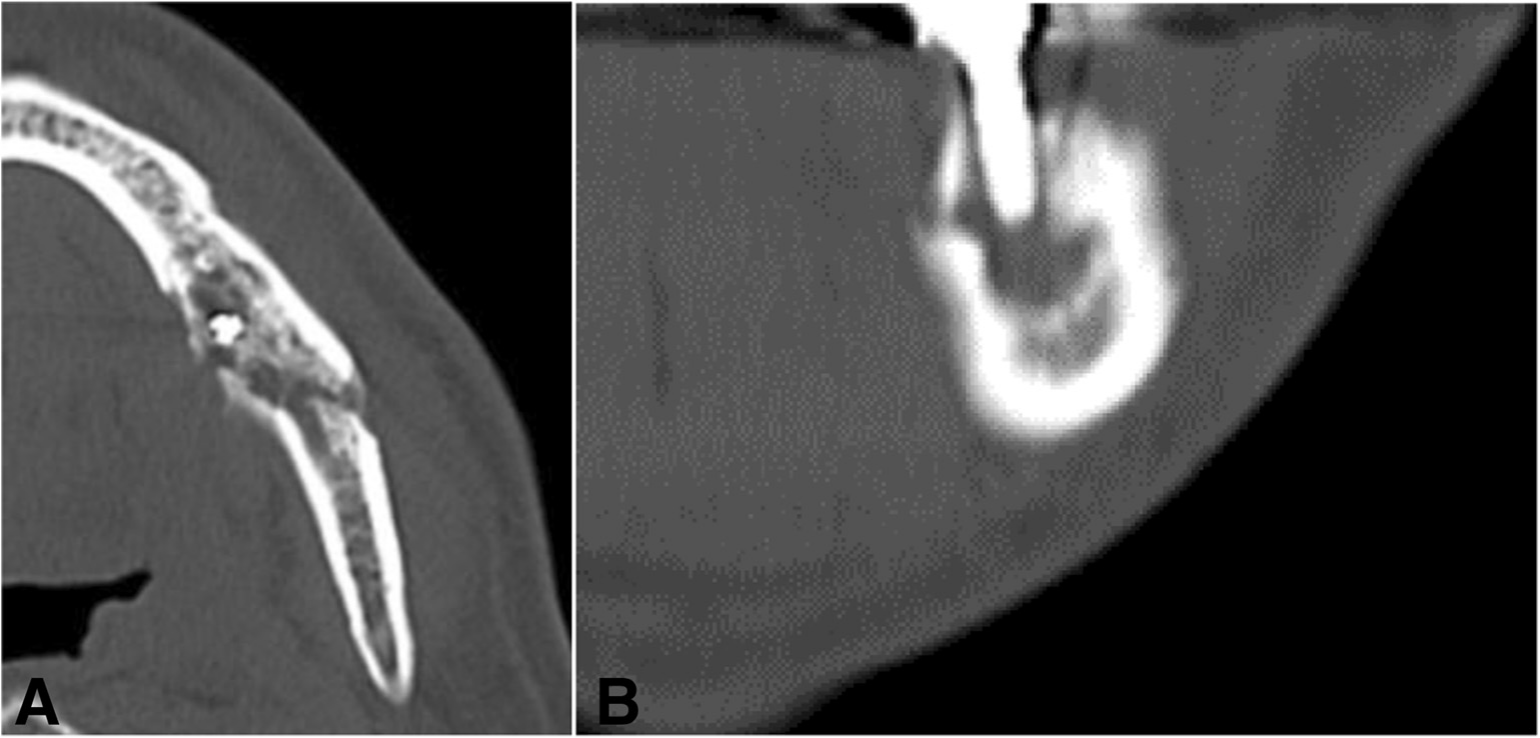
CT images of the left mandible. a Axial view at the left first molar. b Coronal view at the left first molar
